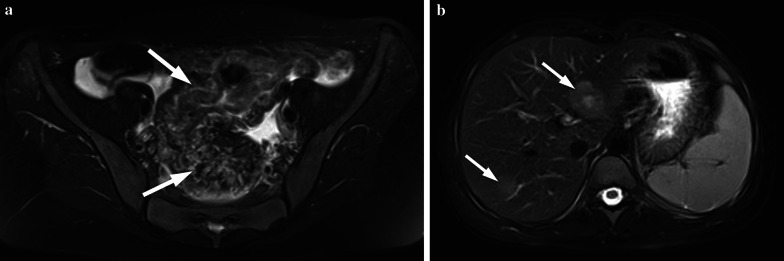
a, b A 14-year-old girl with colon adenocarcinoma. She had blood in her stool and tenesmus for the last 1 month. Hb level was 6.3 gr/dL (normal range 11.7–15.5 gr/dL), Ca 19–9: 539 U/mL (normal range 0–35 U/mL), CeA: 207 ng/mL (normal range 0–7 ng/mL). US showed multiple lesions in the liver with hypoechoic halo. a Axial fat-saturated T2-weighted MRI demonstrates increased wall thickening at the sigmoid and rectal colon (arrows). b Axial fat-saturated T2-weighted MRI shows mild hyperintense liver metastases (arrows). She was operated
